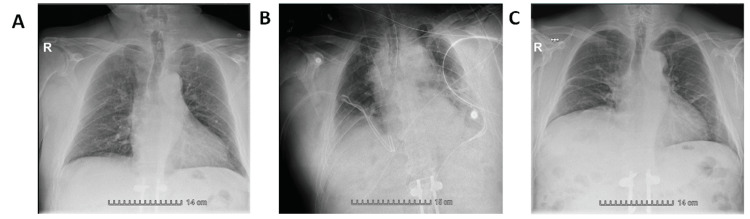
Serial radiographs. Panel A was taken pre-operative, showing normal bilateral diaphragm domes. The RX taken in panel B was taken day 1 postoperative, showing a consolidation of the left lower lobe. The diaphragm is hard to evaluate because of the supine position and positive pressure ventilation. Panel C shows the RX taken a few week after surgery, showing an elevation of the right diaphragm dome.
